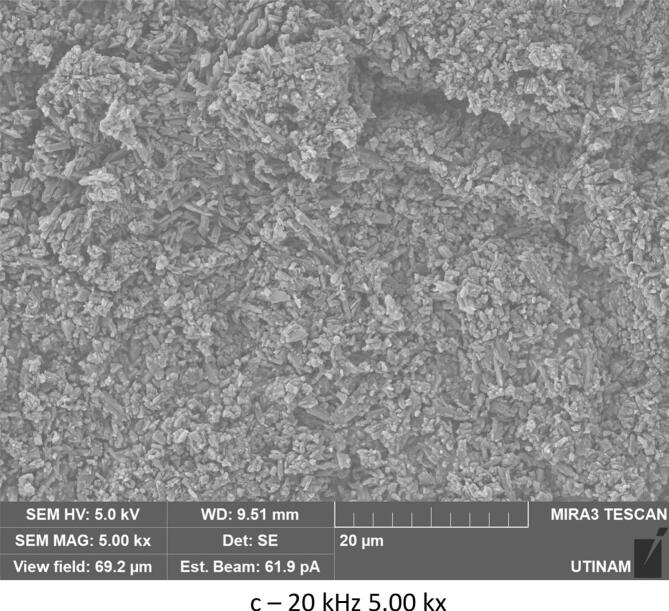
SEM analysis of crystals obtained under different treatments with the same magnification 5.00 kx after 30 min sonication/stirring (tests were carried out using the same concentration of 0.040 g/mL in sodium amoxicillin at pH 4.5 and using a power of around 7.25 W for the ultrasound treatment and a stirring speed of 100 rpm for silent conditions).
